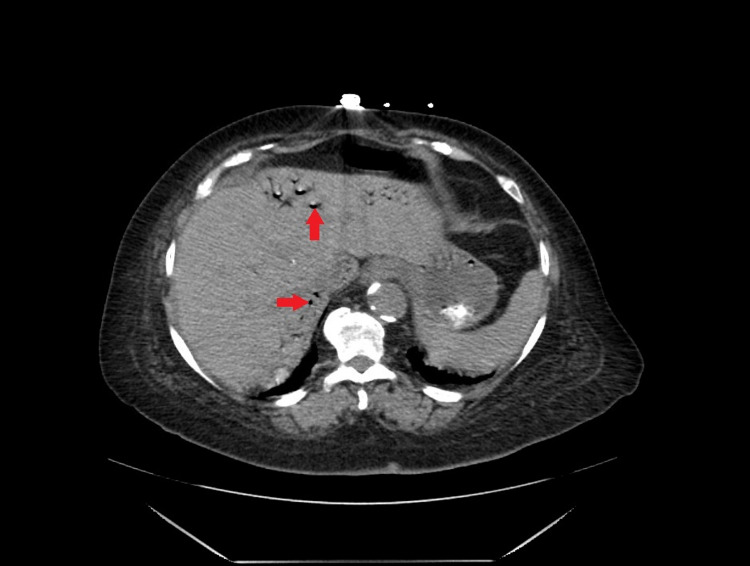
CT scan of abdomen and pelvis; red arrow points to air in portal venous vasculature.
